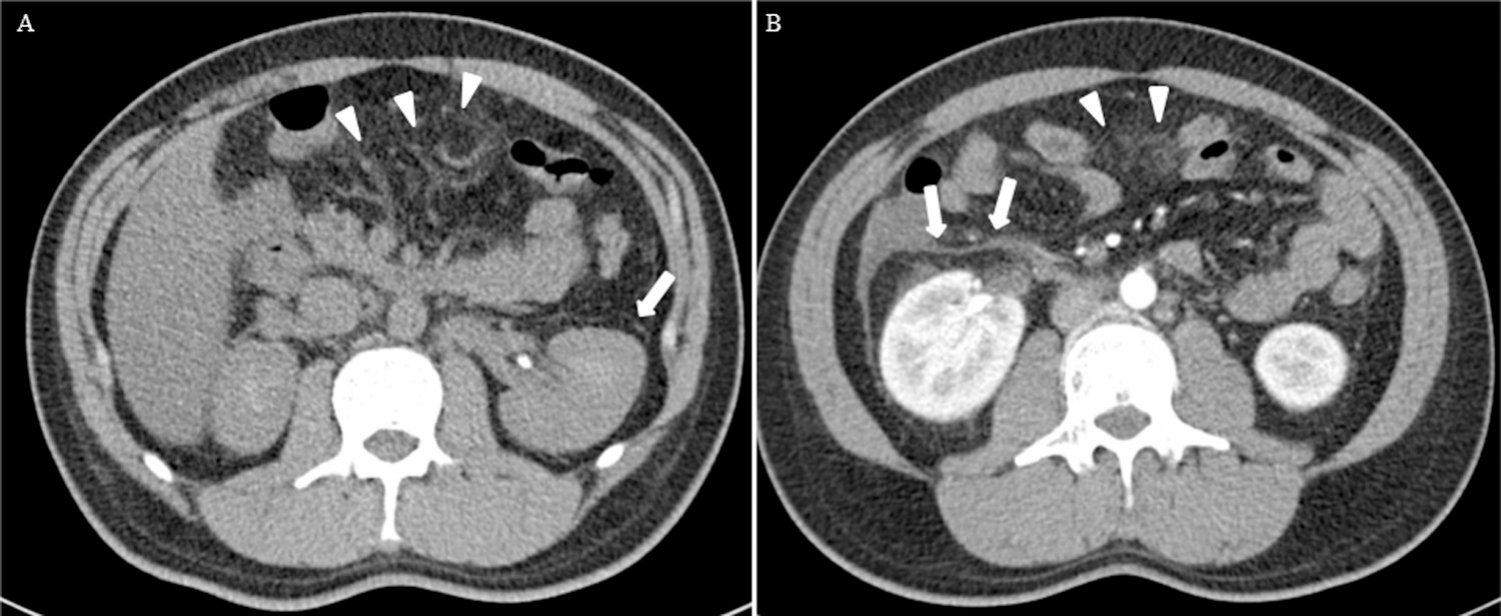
Computed tomography of the abdomen for Case 9. (A) Plain film showing peritonitis (arrowhead) and thickened capsule of the left kidney (arrow). (B) Contrast-enhanced image (arterial phase) showing peritonitis (arrowhead) and thickened capsule of the right kidney (arrow).
